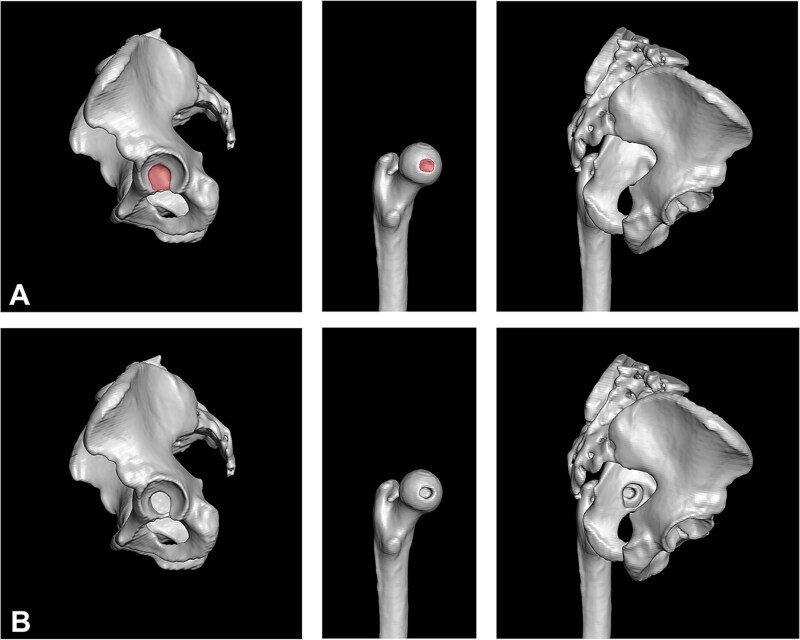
(A and B) The three-dimensional model is built based on the CT scans of the pelvis and femur. (A) The acetabular fossa and fovea capitis are identified. (B) The fossa is excised and the fovea is manually deepened.
